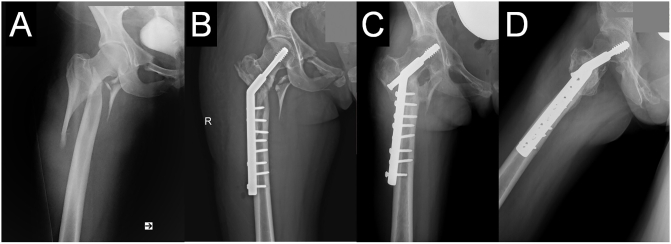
Pre-operative radiograph showing the reverse oblique intertrochanteric AO/OTA 31-A3 fracture (A), postoperative radiograph of the right hip 2 weeks after the initial operation (B) and a radiograph made nine months after the first surgery with visible osteonecrosis of the femoral head and cutout of the lag screw (C, D).
